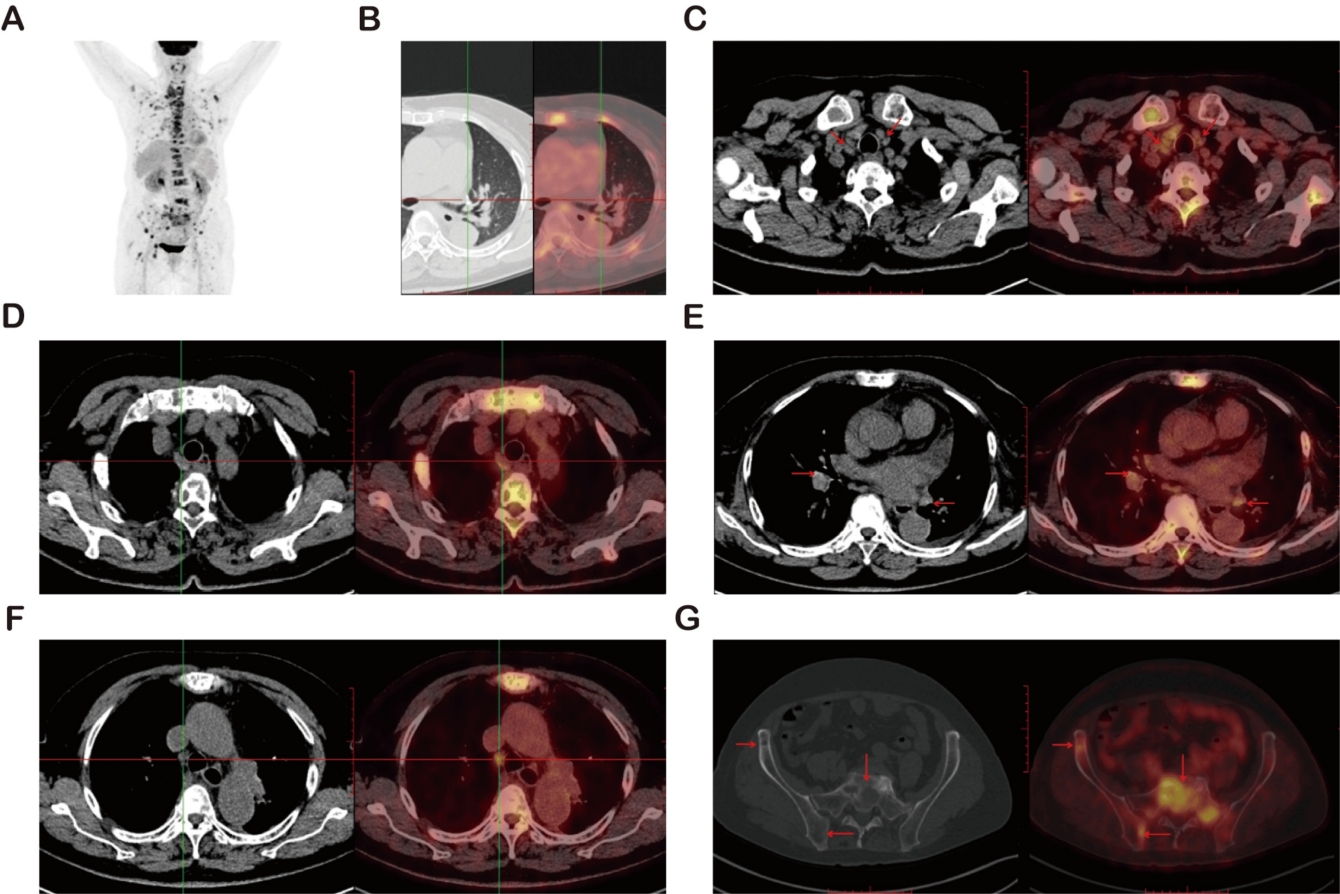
PET-CT Findings Suggestive of Widespread Lymphatic and Skeletal Metastases (A) PET-CT demonstrates multiple hypermetabolic lesions involving lymph nodes and bones throughout the body. (B) No abnormal FDG uptake detected at the previous surgical site. (C) Increased FDG uptake in right paratracheal lymph nodes (SUVmax 4.3). (D) Hypermetabolic activity in upper paratracheal lymph nodes (SUVmax 3.8). (E) Bilateral hilar lymph nodes with elevated FDG uptake (SUVmax 4.5). (F) Marked uptake in lower paratracheal lymph nodes (SUVmax 6.2). (G) High FDG uptake in the sacrum, indicative of osseous metastasis (SUVmax 7.2).
